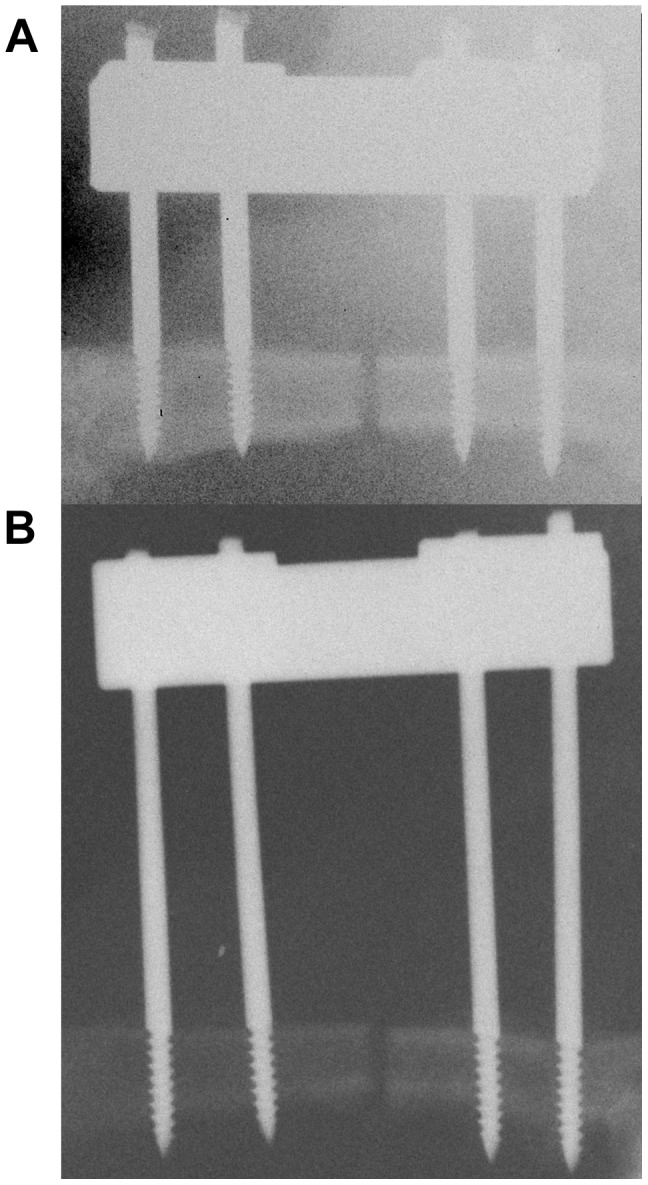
Radiographs of the two fixator configurations varying in the distance between bone and fixator crossbar (offset). (A) Rigid configuration with a 7.5 mm offset. (B) Semi-rigid configuration with a 15 mm offset. Osteotomy gap was set to 1.5 mm.
